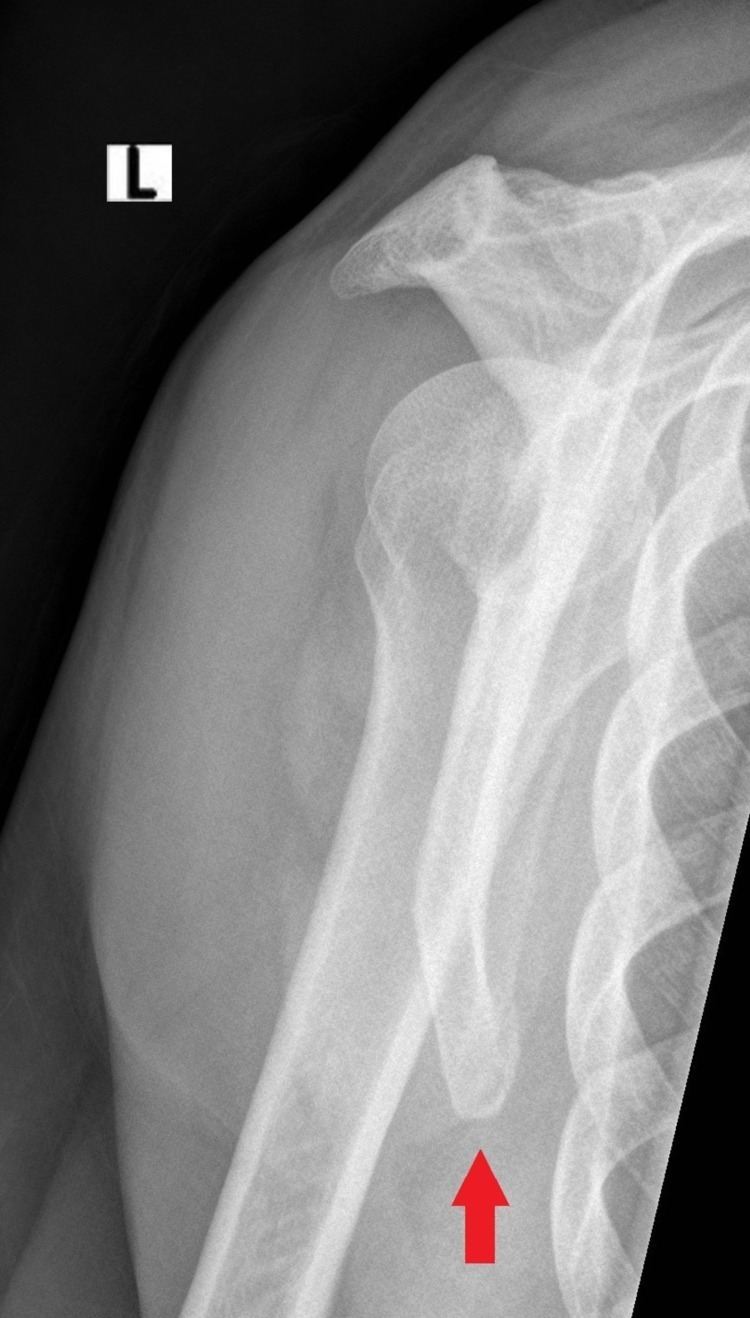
Lateral x-ray at 1.5-month follow-up revealed almost complete fracture healing (red arrow)
